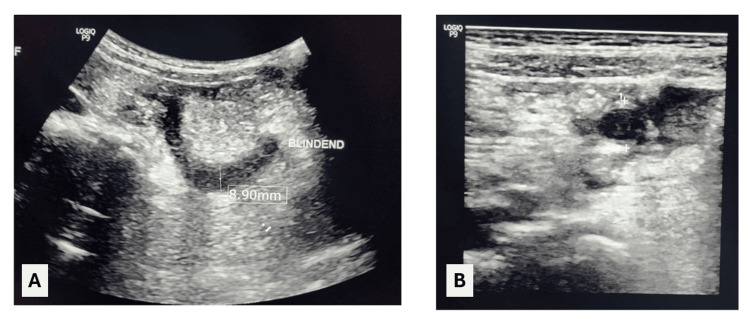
Ultrasonography images suggestive of appendicitis with free fluid
